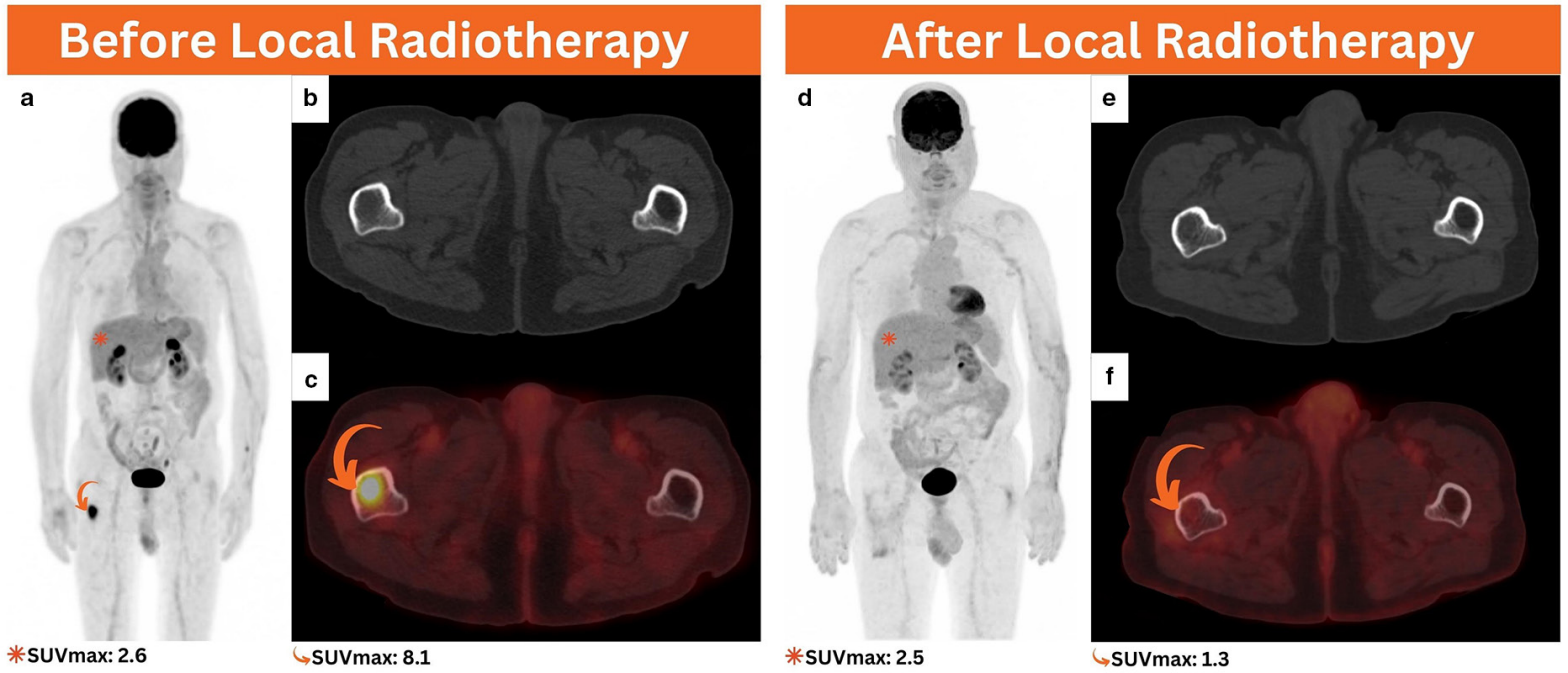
Follow-up ^18^F-FDG PET/CT performed after the initial chemoradiation for response assessment, MIP (a), axial CT (b), and axial PET/CT (c) revealed evidence of a small intramedullary hypermetabolic focus involving the subtrochanteric region of the right femur without significant osseous abnormality on the axial CT images (curved arrows). 3 months following local radiation treatment, ^18^F-FDG PET/CT was performed for response assessment, MIP (d), axial CT (e), and axial PET/CT (f) denoted evidence of a complete metabolic response in the irradiated region (curved arrow). ^18^F-FDG PET/CT, 18-fluorine fludeoxyglucose positron emission tomography/CT; MIP, maximum intensity projection.
